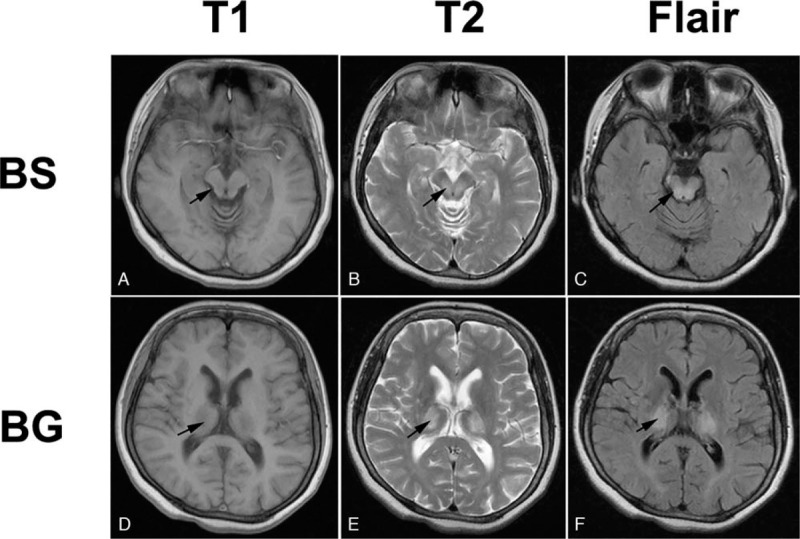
Cranial magnetic resonance imaging image of the patient. Symmetrical patchy signals were observed in brainstem and bilateral basal ganglia in T1-weighted image (A, D), T2-weighted image (B, E), and Flair images (C, F). BS = Brainstem, BG = basal ganglia.
